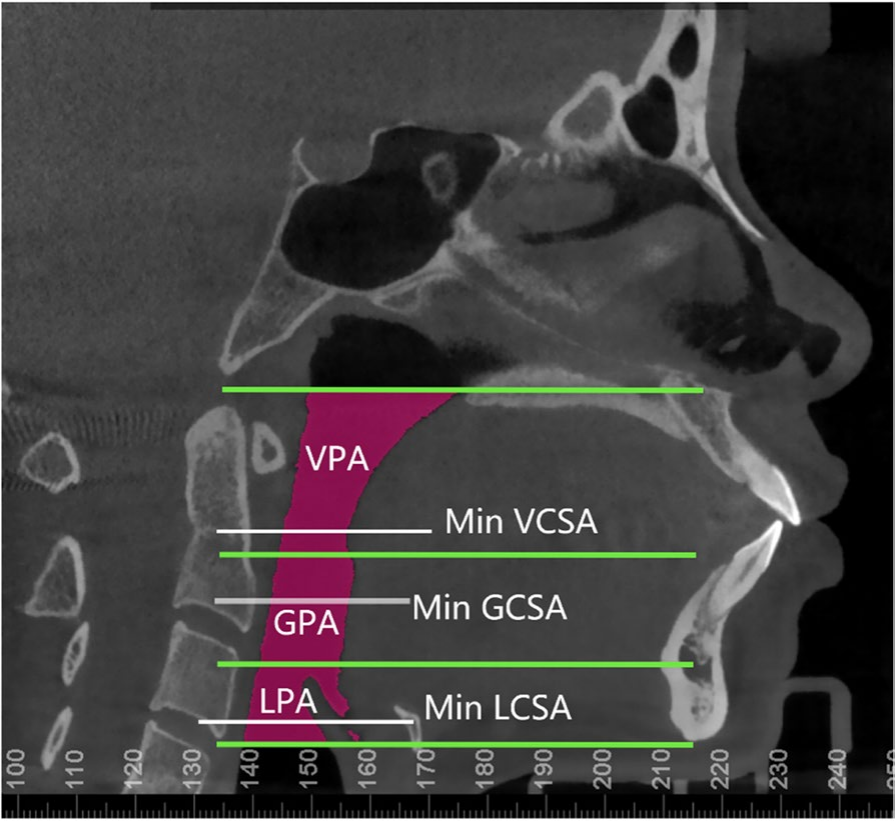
Segments of the upper airway on midsagittal CBCT image using Dolphin Imaging 11.95 software (Chatsworth, Los Angeles, CA, USA). Velopharynx airway (VPA): from the plane of ANS-PNS to the plane across the most posteroinferior point of the uvula; Glossopharynx airway (GPA): from the plane across the most posteroinferior point of the uvula to the plane across the most superior point of the epiglottis. Laryngopharynx airway (LPA): from the plane across the most superior point of the epiglottis to the plane across point of the epiglottic vallecula. Minimum cross-sectional area of each upper airway segment (Min VCSA, Min GCSA and Min LCSA) was automatically identified and measured by the Dolphin software
